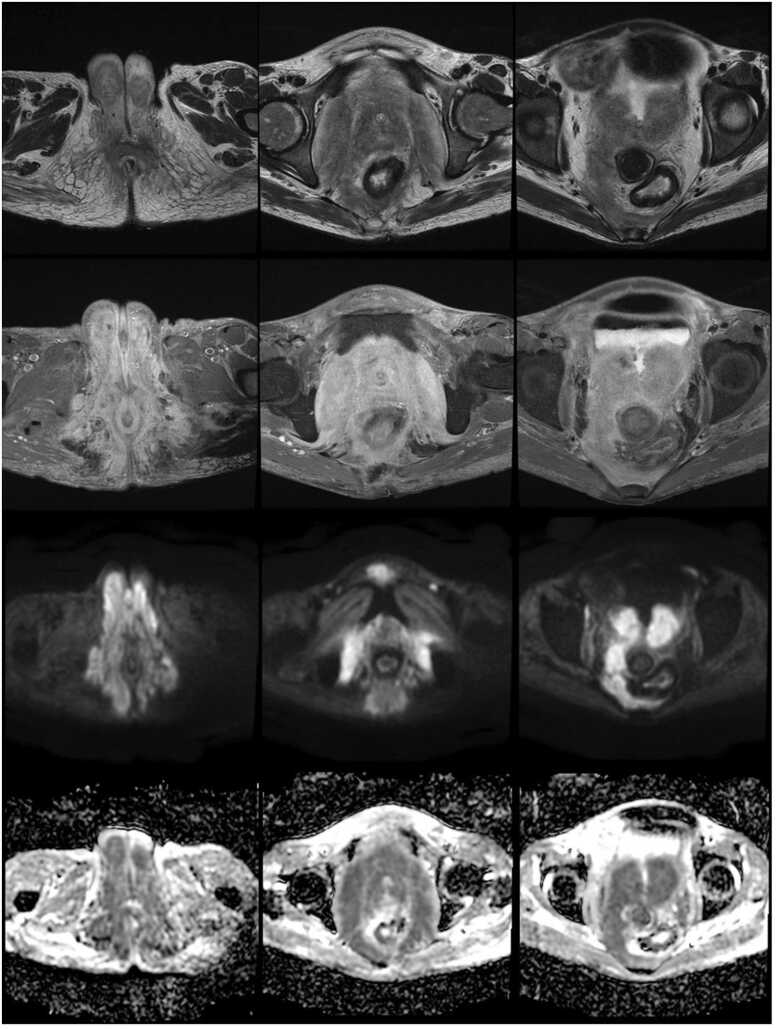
An infiltrative soft tissue is seen in the pelvic and perineum. It infiltrates the perineal skin and both labia major, and bilateral internal obturator muscles leading to the enlargement of these structures. presacral fat, and mesorectal fascia in the right side and the bladder neck were also involved. The lesion had an iso signal on T1 weighted, and a mildly high signal on T2 weighted images, showing mild enhancement and restriction of diffusion on DWI sequences. (from top to bottom first row: T2 weighted, second row: T1 weighted with contrast,third row: DWI and the fourth row:ADC).
